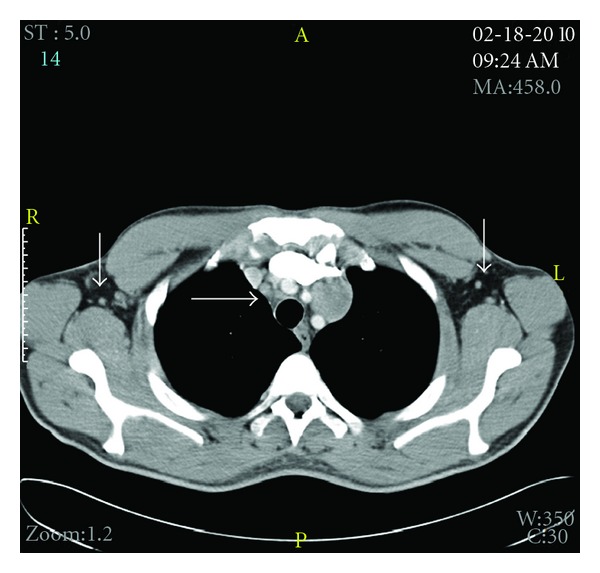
CT chest showing mediastinal lymphadenopathy and bilateral axillary enlarge lymph nodes.
